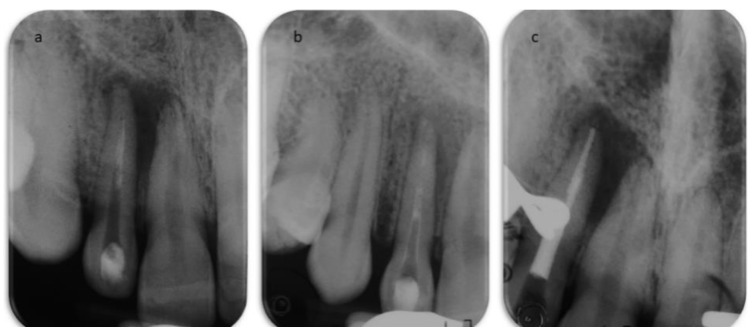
Root canal treatment. (a) Periapical radiograph after the first access to the root canal system. (b) Radiograph showing the calcium hydroxide temporary dressing within the canal after the first visit. (c) Periapical radiograph after obturation of the root canal system.
